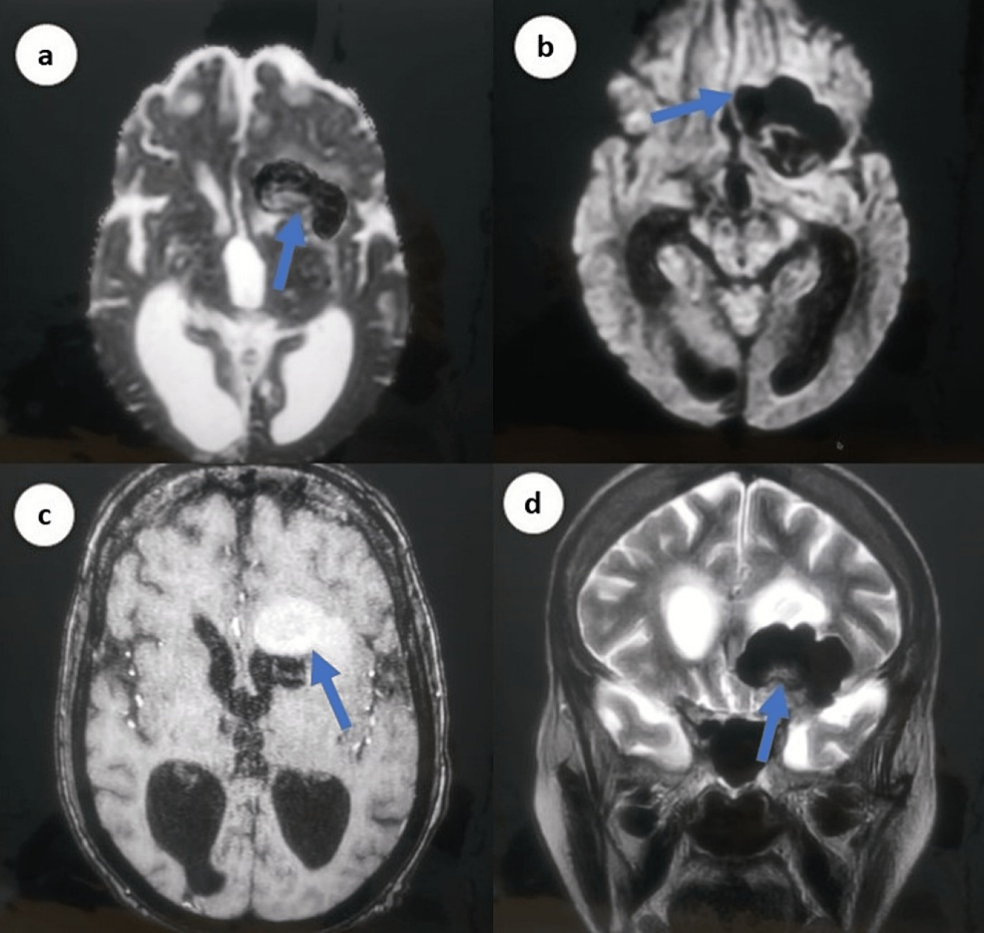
Brain MRI demonstrating a high-intensity cystic lesion in the middle and anterior cranial fossa with thin marginal enhancement and calcifications and area of gliosis was visualized in the right capsular, periventricular regions, and right occipital region with ex vacuo dilatation of the occipital horn of the right lateral ventricle: (a) FLAIR, (b) DWI), (c) T1, (d) T2 MRI: magnetic resonance imaging, DWI: diffusion-weighted imaging, FLAIR: fluid attenuated inversion recovery
